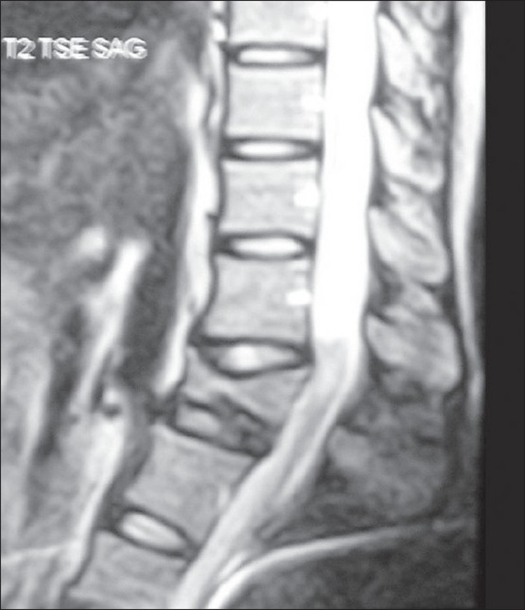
Mid sagittal T2-weighted image of MRI of dorso-lumbar spine shows the post-traumatic khyphosis at the L1 level with syringomyelia
